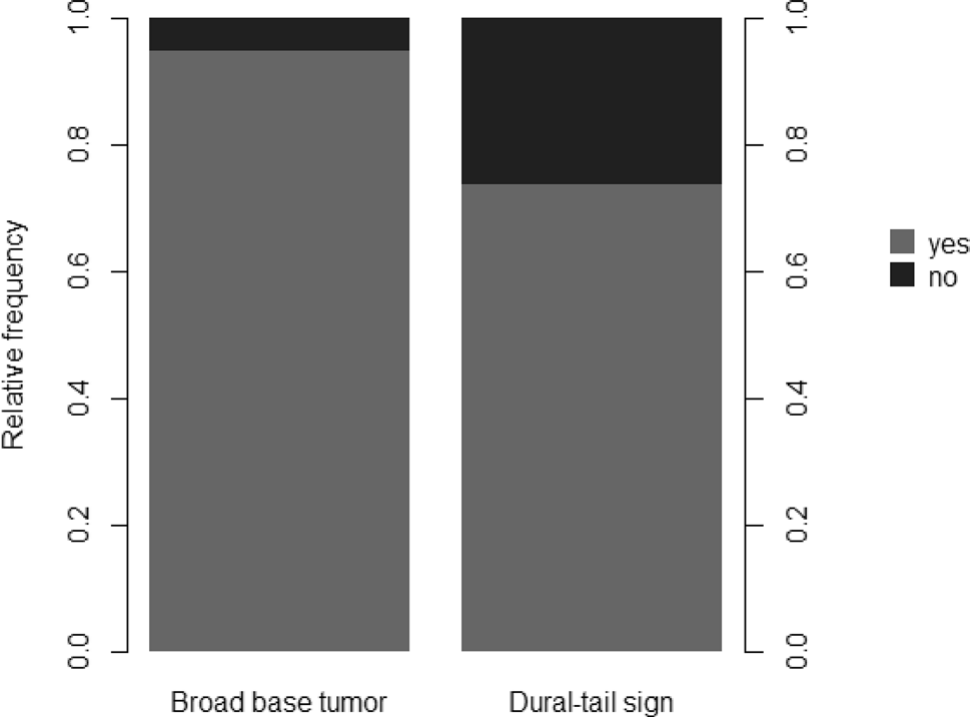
Broad-based tumor and dural tail sign as meningioma MRI-characteristics. Typical structural aspects for meningioma were mainly and easily identified based dural tail sign and its broad base. Other features such as hypointensity in T1 or hyperintensity in T2 to grey matter or calcifications were less often.
